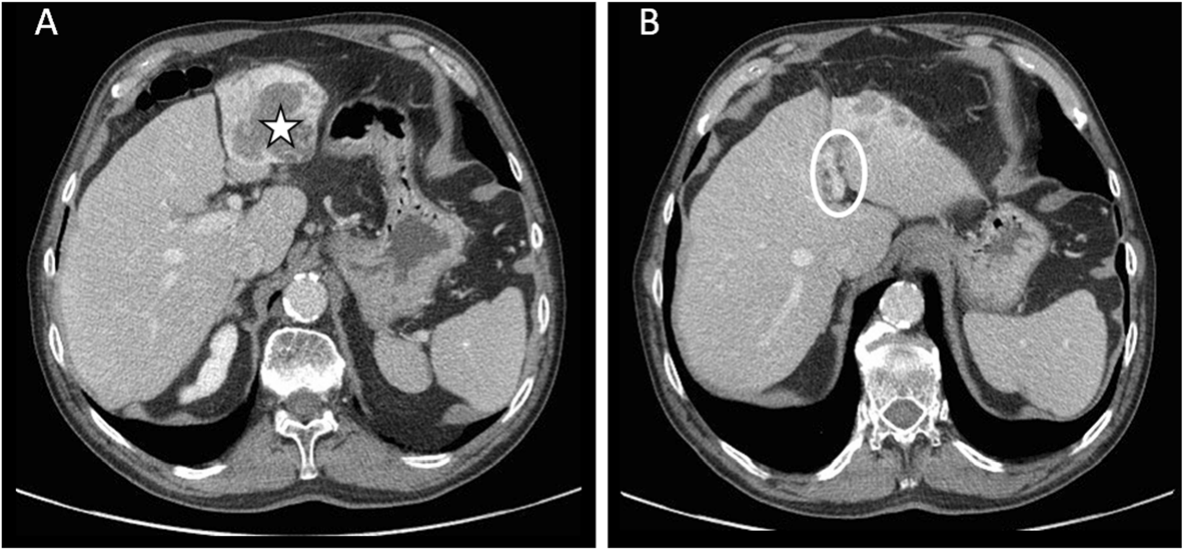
Computerized tomography (CT) scan of the left liver tumor lesion after 9 months of treatment with sorafenib. (A) Left liver tumor lesion (star), and (B) regression of the lesion with recanalization of the left portal branch (circle). CT, computerized tomography.
